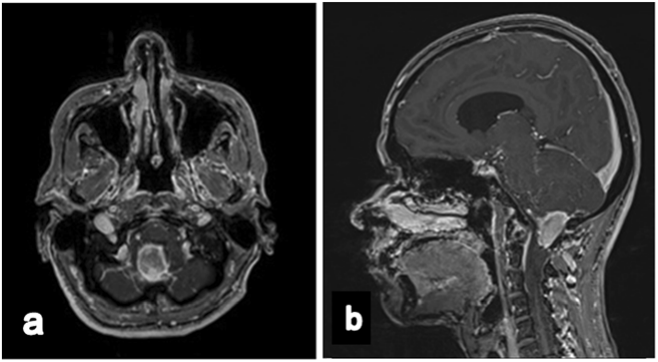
Preoperative (a) axial and (b) sagittal T1-weighted magnetic resonance imaging after gadopentetate dimeglumine application, demonstrating a well-defined enhancing mass located dorsal from the medulla oblongata with brainstem attachment.
